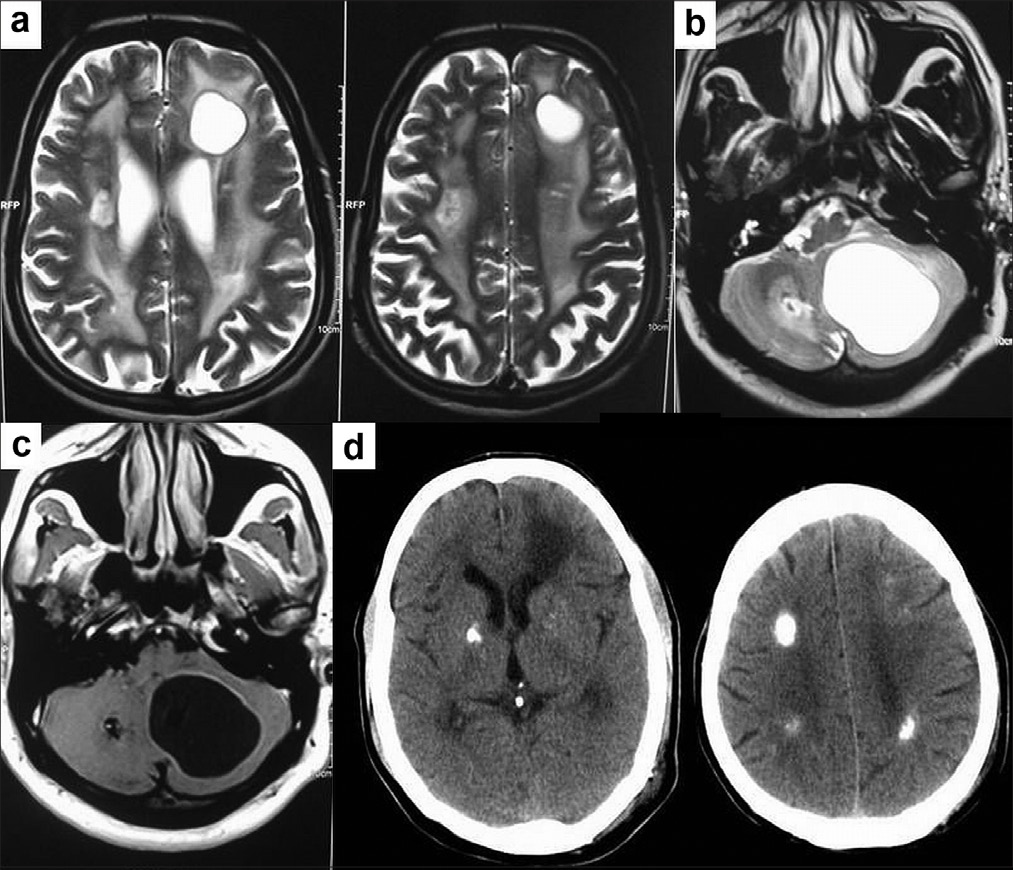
(a) T2-weighted (T2W) magnetic resonance imaging (MRI) of brain: bilateral cystic lesions and diffuse white matter lesions; (b) a large right cerebellar cyst compressing the 4th ventricle and brainstem; (c) T1W MRI brain after intravenous gadolinium enhancement of cyst wall; and (d) computed tomography of brain showing multiple calcifications
